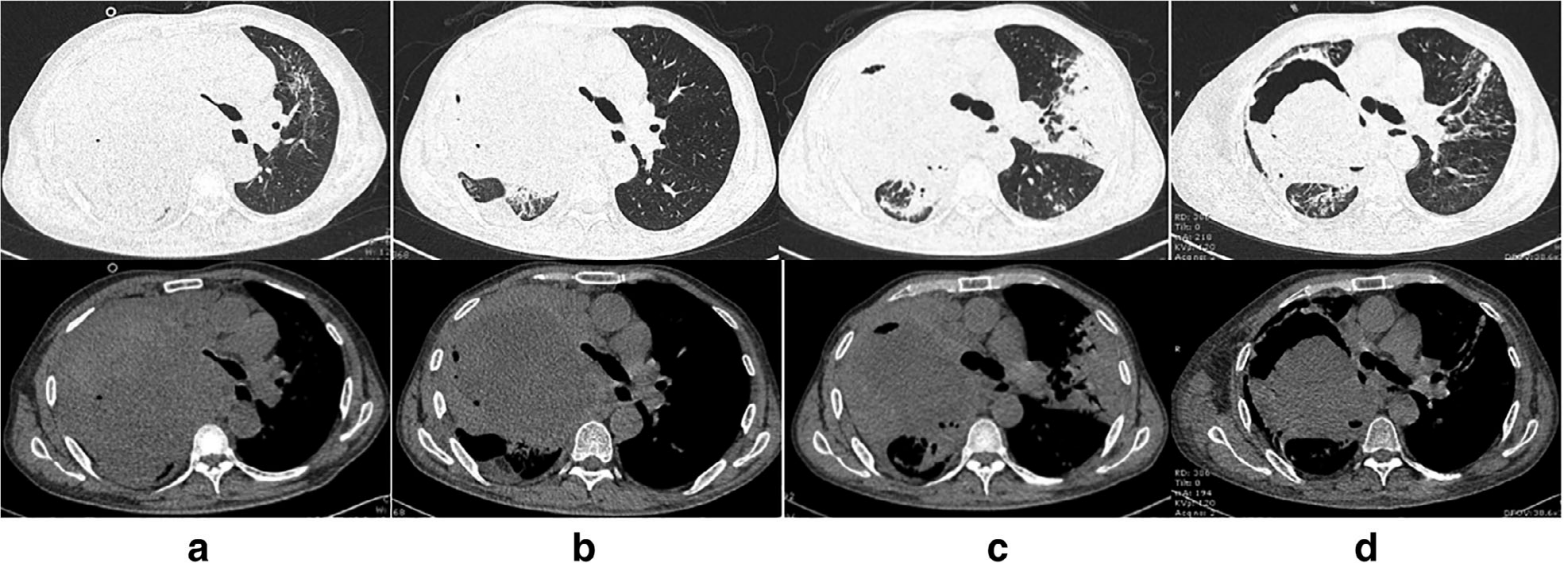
Thoracic computed tomography (CT) scan at different times. (a) A giant mass lesion was visible in the right lung with obvious compression of the trachea and esophagus before treatment with pembrolizumab (30 July 2019). (b) A shrinkage was seen in the size of the mass with a large low density area and significant compression release of trachea and esophagus after four cycles of pembrolizumab (29 October 2019). (c) Consolidation was seen in the left lung and small transparent area within the mass lesion in right lung after eight cycles of pembrolizumab (31 January 2020). (d) Cavitation in the lesion in the right lung, while consolidation in the left lung absorbed after methylprednisolone treatment (20 February 2020).
